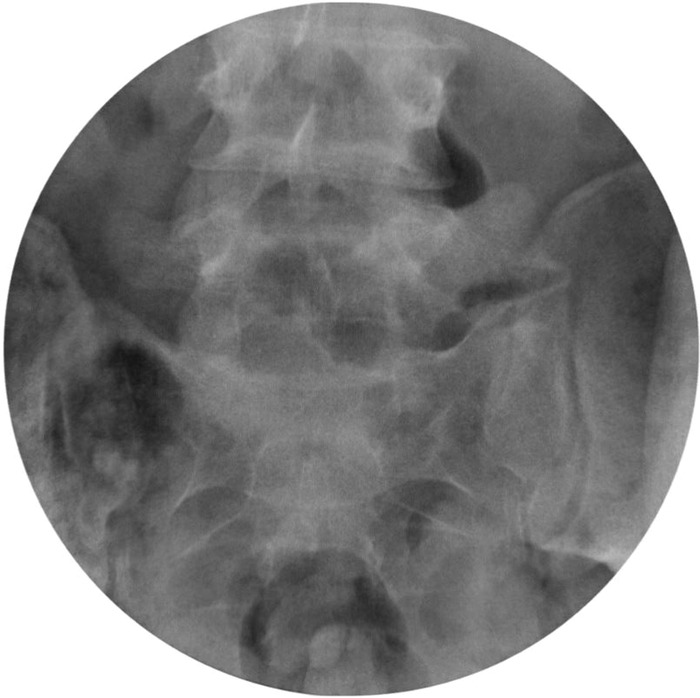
Anteroposterior lower lumbar spine intraoperative radiograph demonstrates excision of L5 transitional articulation with the sacrum on the left.
